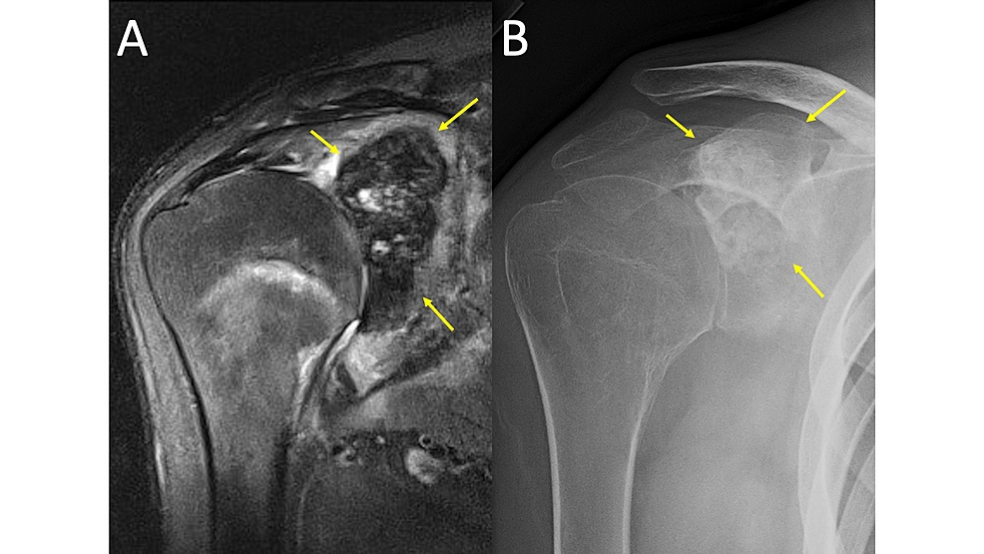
Chondroblastoma MRI coronal T2 fat saturation (FS) shoulder (13A) demonstrates a mildly heterogeneous, predominately hypointense, lobulated mass within the glenoid (yellow arrows). There is glenohumeral joint effusion, synovitis, and surrounding soft tissue and bone marrow edema. The imaging appearance suggests an aggressive process. A Grashey view radiograph of the right shoulder (13B) demonstrates a lobulated, sclerotic mass (yellow arrows) with an “arc and ring” appearance. Incidentally, there is severe glenohumeral joint space loss new from comparison radiographs (not shown) likely related to chronic inflammation of the glenohumeral joint. The location of the lesion and radiographic appearance is most consistent with chondroblastoma. The radiographic appearance is less aggressive than MRI findings.
